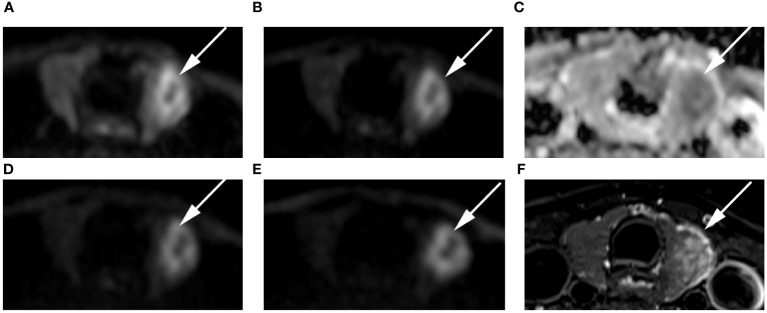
Images of a 54-year-old female with left lobe papillary carcinoma (arrow): DWI image with a b-value of 500 s/mm^2^
